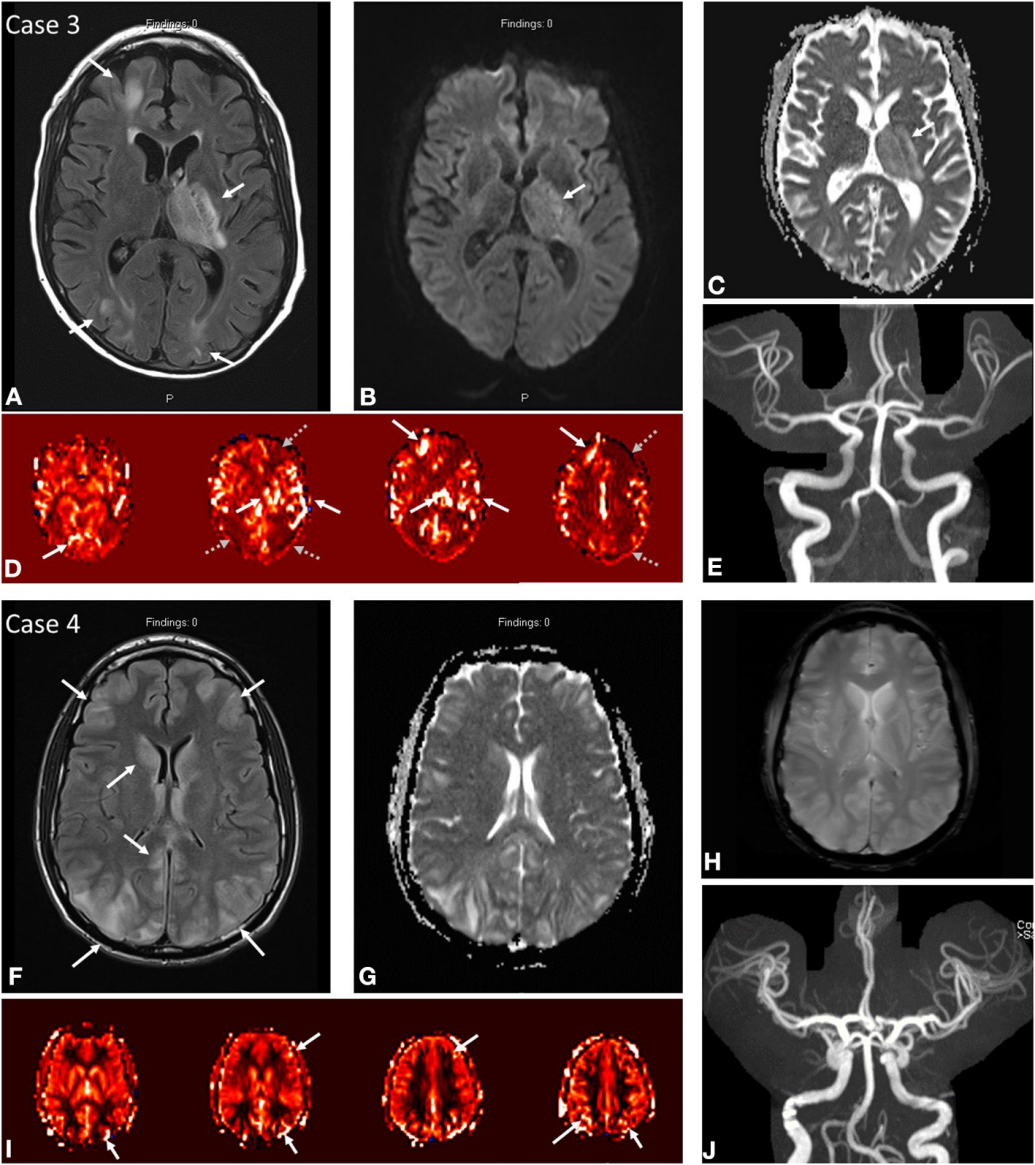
Case 3: A 53-year-old male presenting with seizure activity and past medical history notable for EtOH cirrhosis status post recent liver transplant on tacrolimus complicated by post-operative AKI on intermittent HD. MRI brain at initial presentation demonstrates abnormal FLAIR hyperintensity (A) in the left thalamus, posterior limb of the left internal capsule, and cortex/subcortical white matter of the left frontal and bilateral occipital lobes. DWI (B) and ADC (C) demonstrate predominantly T2 shine through in the left thalamus/internal capsule. ASL (D) demonstrates increased signal in the corresponding territories (solid white arrows) with notable severe border-zone hypoperfusion in the bilateral watershed territories (gray dashed arrows). No focal arterial high-grade stenosis or occlusion on MR angiography (E). Case 4: A 21-year-old male presenting with seizure activity in setting of hypertension of 177/113 with past medical history notable for thrombocytopenia, oliguric renal failure on intermittent HD secondary to atypical hemolytic uremic syndrome, hemophagocytic lymphohistiocytosis on etoposide/steroids, and prior history of PRES. MRI brain demonstrates abnormal FLAIR hyperintensity (F) in the cortex and subcortical white matter of the bilateral frontal lobes and bilateral parieto-occipital regions with slight asymmetric signal hyperintensity in the right caudate nucleus. ASL (I) demonstrates increased signal in the corresponding bilateral frontal, parietal, and occipital regions. No DWI/ADC (G) or SWI (H) signal abnormality. No focal arterial high-grade stenosis or occlusion on MR angiography (J).
